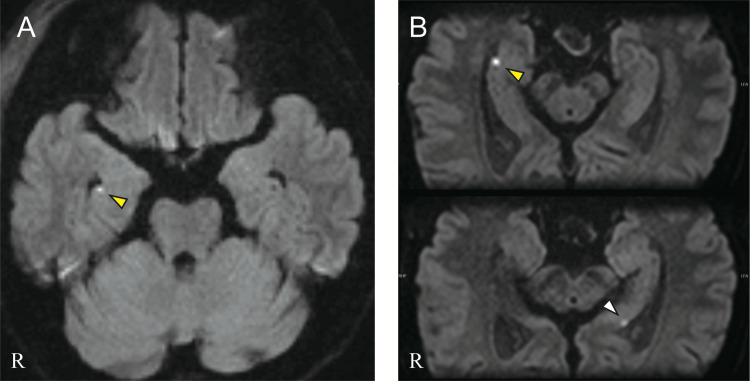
Diffusion-weighted imaging (DWI) showing punctate hippocampal lesions A: On the second day of hospitalization, DWI with 5 mm slices demonstrated a punctate hyperintensity in the right hippocampus (yellow arrowhead). B: On the third day, DWI performed parallel to the long axis of the hippocampus with 3 mm slices detected the previously observed punctate hyperintensity in the right hippocampus (yellow arrowhead) and a similar finding in the left hippocampus (white arrowhead). Both lesions were located in the CA1 regions.
